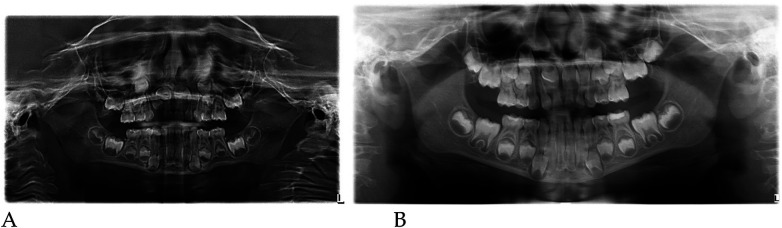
Pre- (A) and post-treatment (B) panoramic radiographs of case 8.
